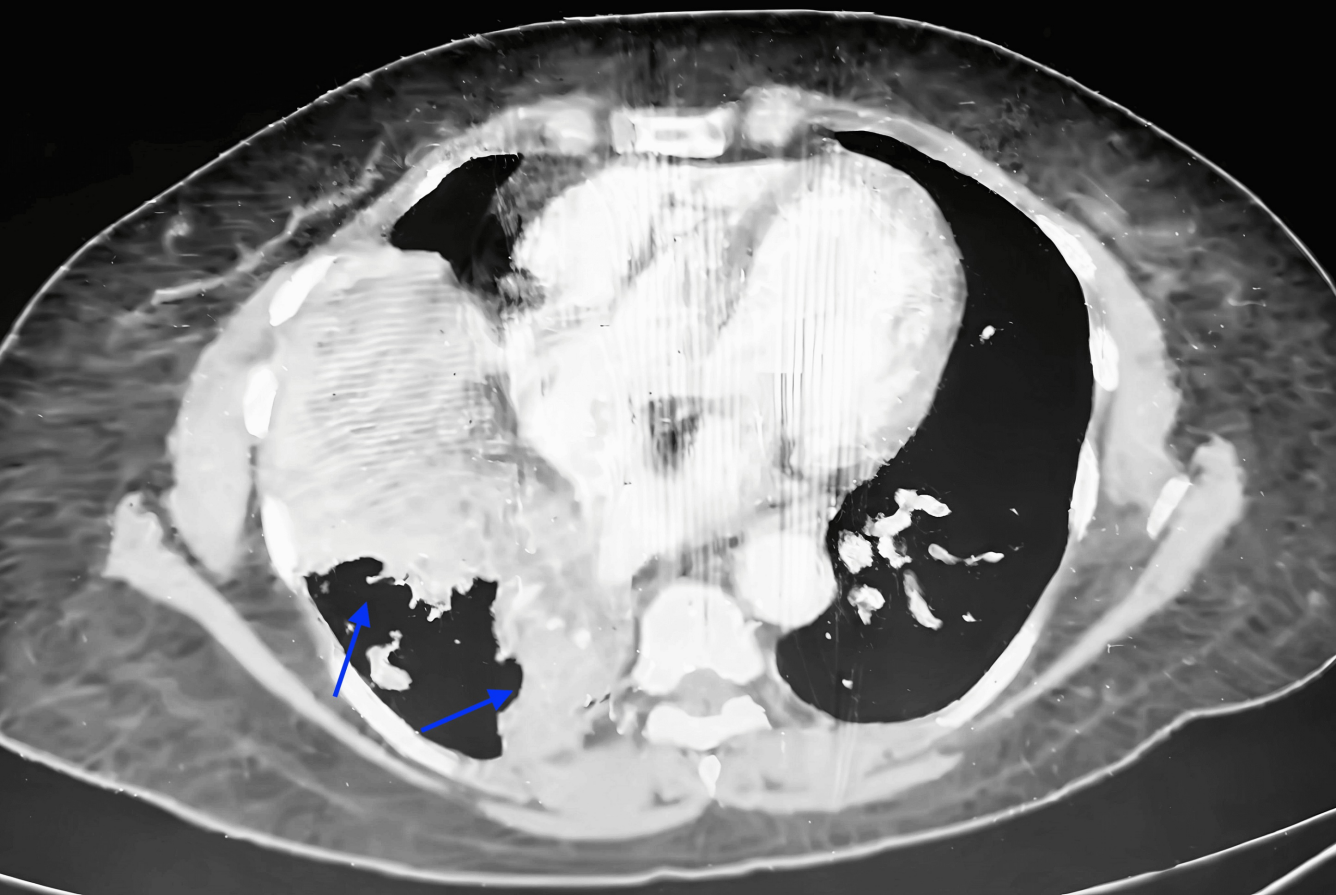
Axial CT of the thorax showing partial collapse of the right lower lobe secondary to endobronchial obstruction (blue arrows) CT, Computed Tomography
